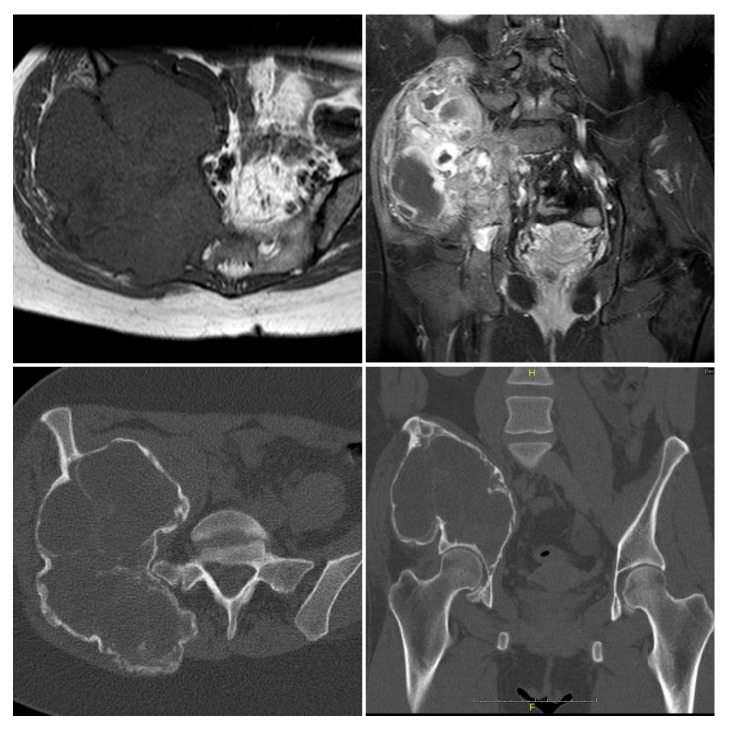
Initial MR and CT Imaging showing a substantial right-sided cystic tumor of the pelvis infiltrating associated neuroforamina.
